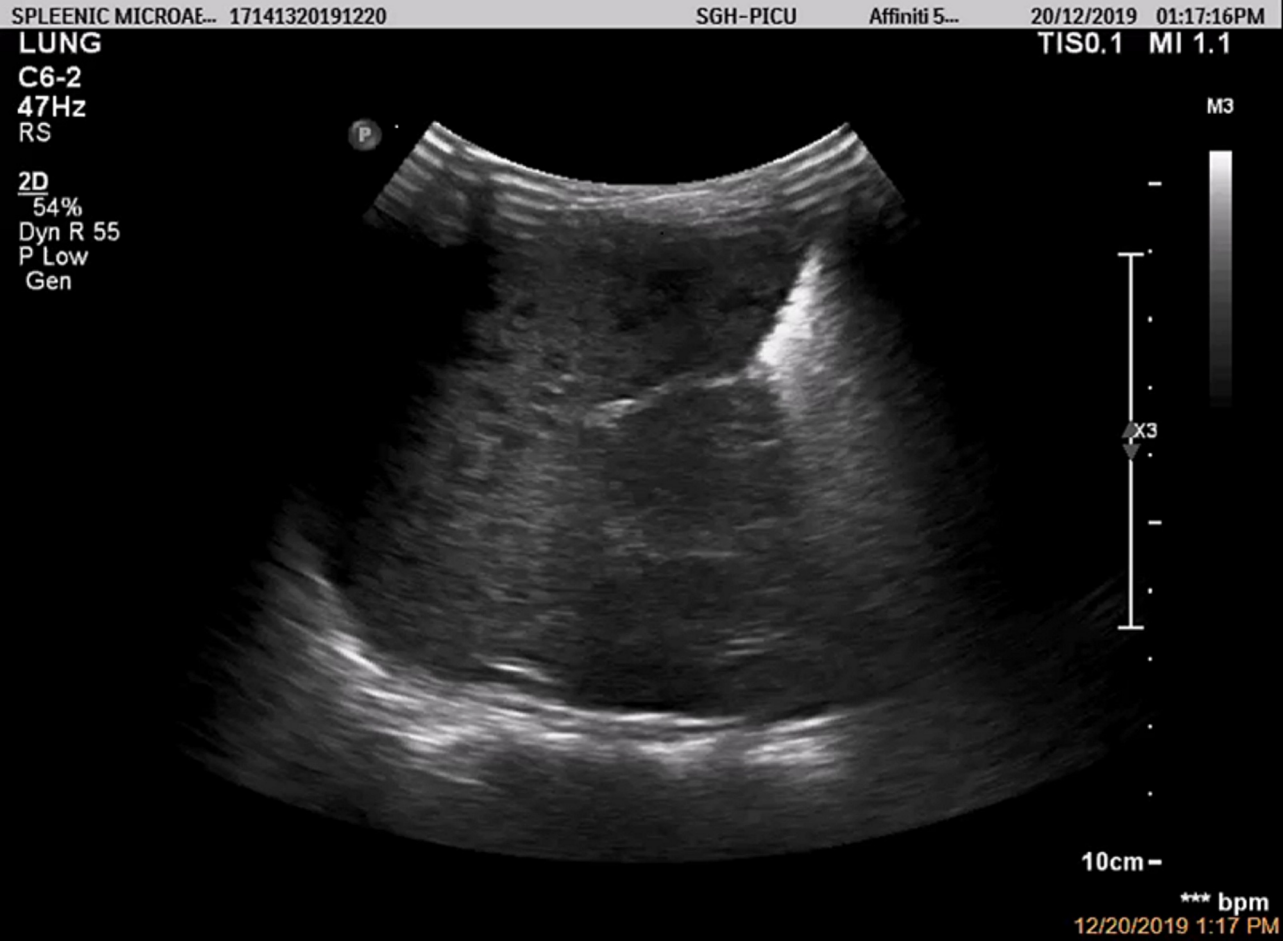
Multiple splenic abscesses seen on the point-of-care ultrasound using a curvilinear transducer.
